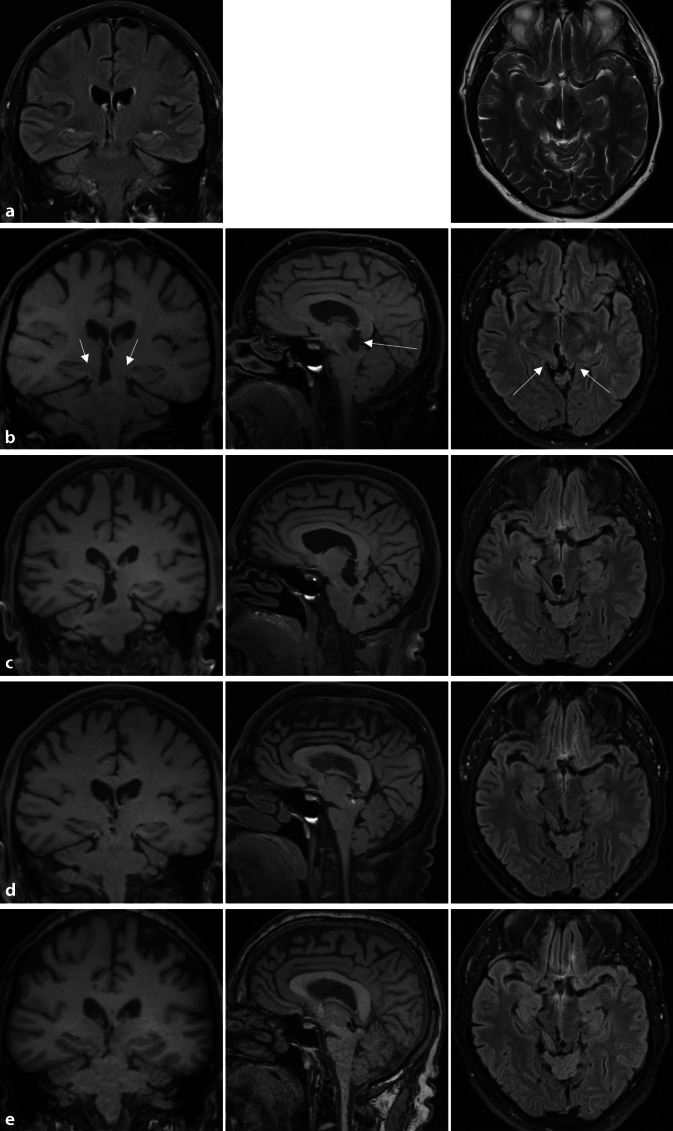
Kraniale Bildgebungen (MRT) im zeitlichen Verlauf: exemplarische cMRT-Bildgebungen koronar (*erste Spalte*), sagittal (*zweite Spalte*) und transversal (*dritte Spalte*). a Externe Bildgebung, 08/2023 (koronar FLAIR, axial T2): Kaudalverlagerung der Mittellinienstrukturen, kleiner zystischer Defekt mit Flüssigkeitsansammlung um den ehemaligen Iod125-Seed, Hernierung des Hippokampusgewebes. b 11/2023, T1 koronar: weiterhin Kaudalverlagerung der Mittellinienstrukturen, Hernierung der temporomesialen Strukturen beidseits (*exemplarisch Pfeile*). T1 sagittal: zunehmende zystische Läsion mesenzephal (*Pfeil*), Kaudalverlagerung Pontomesenzephalon, Kleinhirntonsillentiefstand, T2 FLAIR transversal: zystische Läsion mesenzephal, Hernierung der Hippokampusstiele am Tentorium cerebelli (*exemplarisch Pfeile*). c 03/2024: Größenzunahme der mesenzephalen zystischen Läsion mit Verdrängung des Pons bei weiter bestehender Kaudalverlagerung der Mittellinienstrukturen. d 05/2024, T1 koronar/sagittal sowie T2 FLAIR transversal: nach Anlage des Ommaya-Reservoirs deutlich regrediente zystische Läsion mesenzephal, weiterhin Kaudalverlagerung der Mittellinienstrukturen mit Herniation der Hippokampusstiele bds. e 11/2024, MPRAGE koronar/saggital sowie T2 FLAIR transversal: im Vergleich zu Mai 2024 unveränderter Befund
